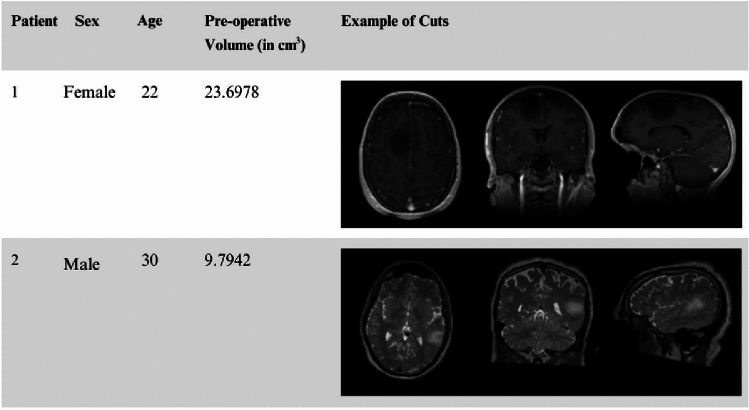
Sample demographic and imaging data from the ReMIND brain tumor MRI dataset^20^ used in the XR application.
